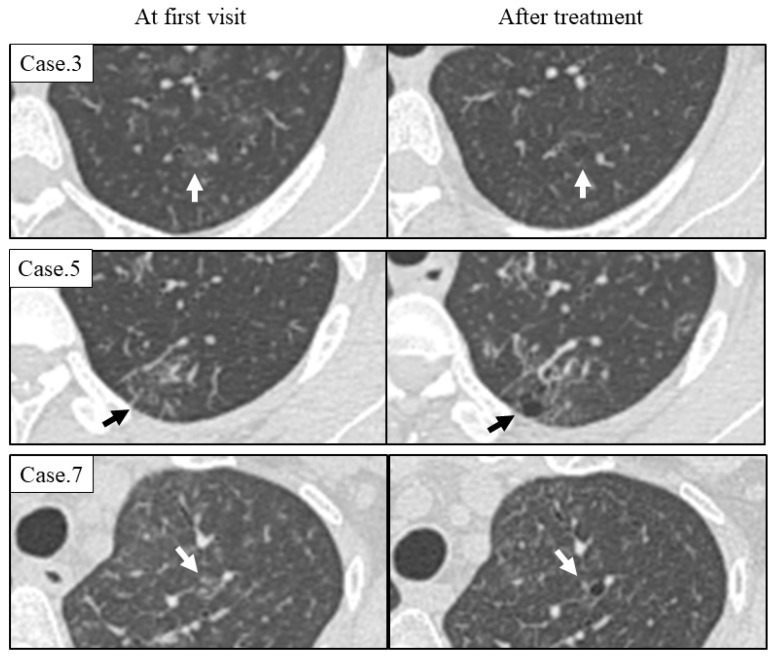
Chest high-resolution computed tomography imaging of cases with newly emerged cysts during the course. A new cyst emerged from the site of ground-glass attenuation on HRCT at the time of initial diagnosis (arrow). The cyst appeared despite the improvement of the ground-glass attenuation with treatment.
